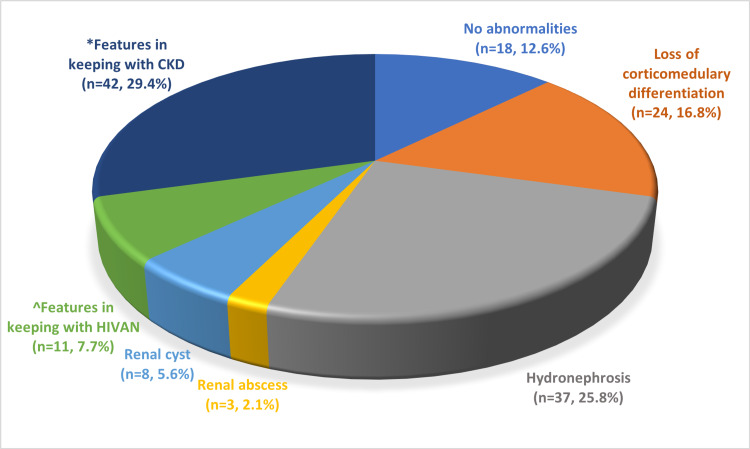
Findings of the 143 subjects in whom renal ultrasonography was performed *Small and hyperechoic kidneys ^Large and hyperechoic kidneys in subjects with HIV
